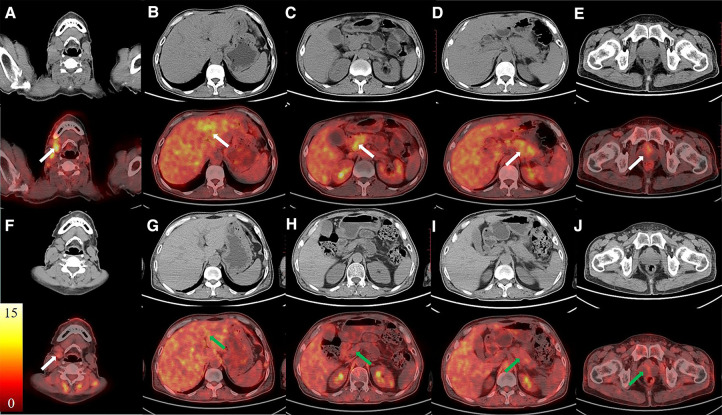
The images of ^18^F-FDG PET/CT. The CT images were in the first/third rows, and the fused PET/CT images were in the second/fourth rows. The pre-treatment images (A–E, white arrows) showed elevated uptake of the following lesions: cervical lymph nodes, intrahepatic bile ducts, pancreas, and prostate. FDG-avid lesion was detected only in the right cervical lymph node after the treatment (F–J).
